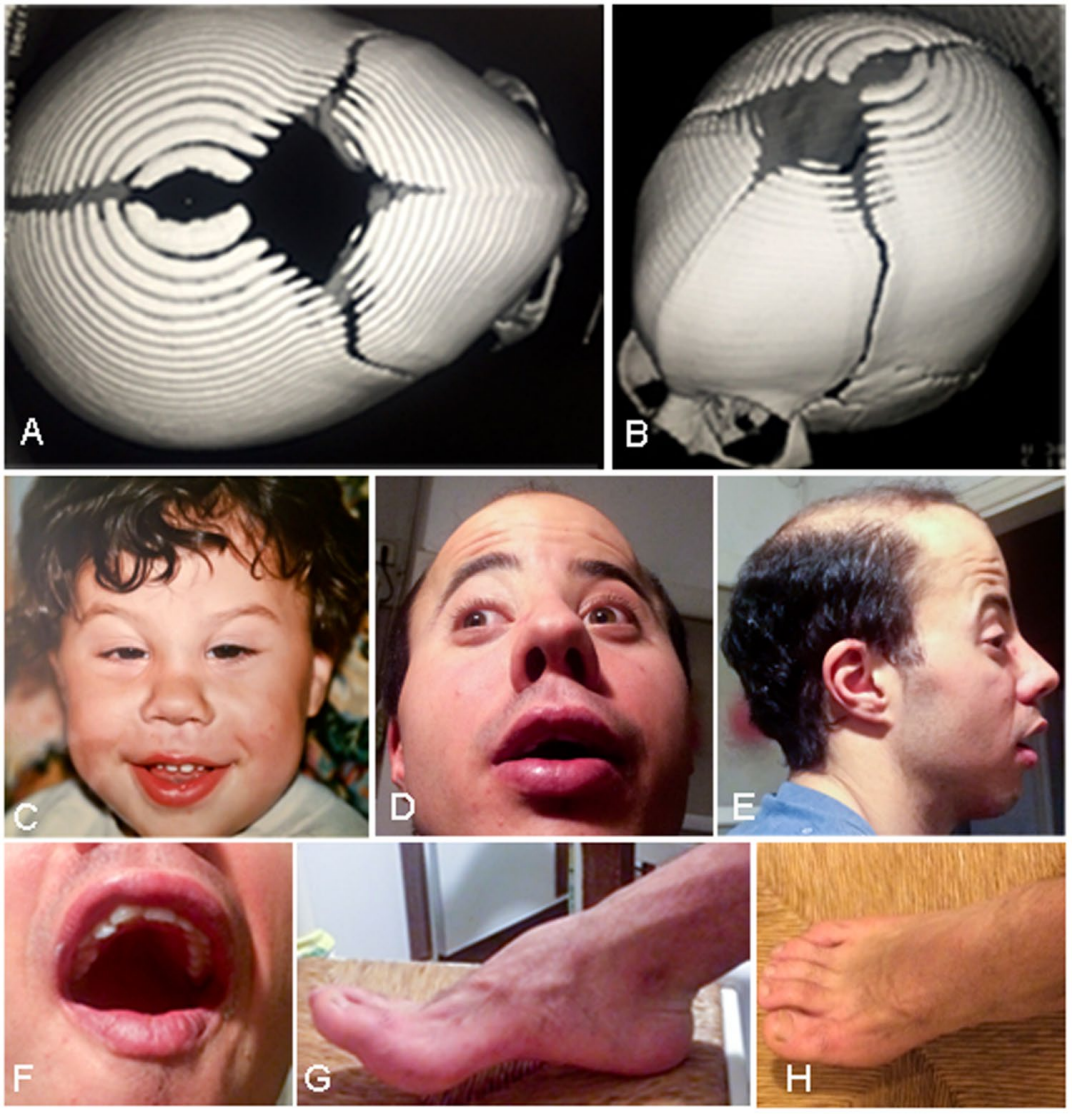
Main features of the patient. (A–B) Neonatal CT scan. Trigonocephaly is clearly appreciated. For a control CT scan, see Khanna *et al*.^32^ (C–E) Facial dysmorphisms at 2 and 23 years of age. Hypertelorism, convergent strabismus, down slanted palpebral fissures, epicanthus, prominent nasal root and ante-verted nostrils and (F) arched palate and hypertrophy of the alveolar processes are appreciated. (G–H) Foot phenotype with metatarsal flat-footedness and clinodactyly. All images are shared by the family with explicit permission to publish.
